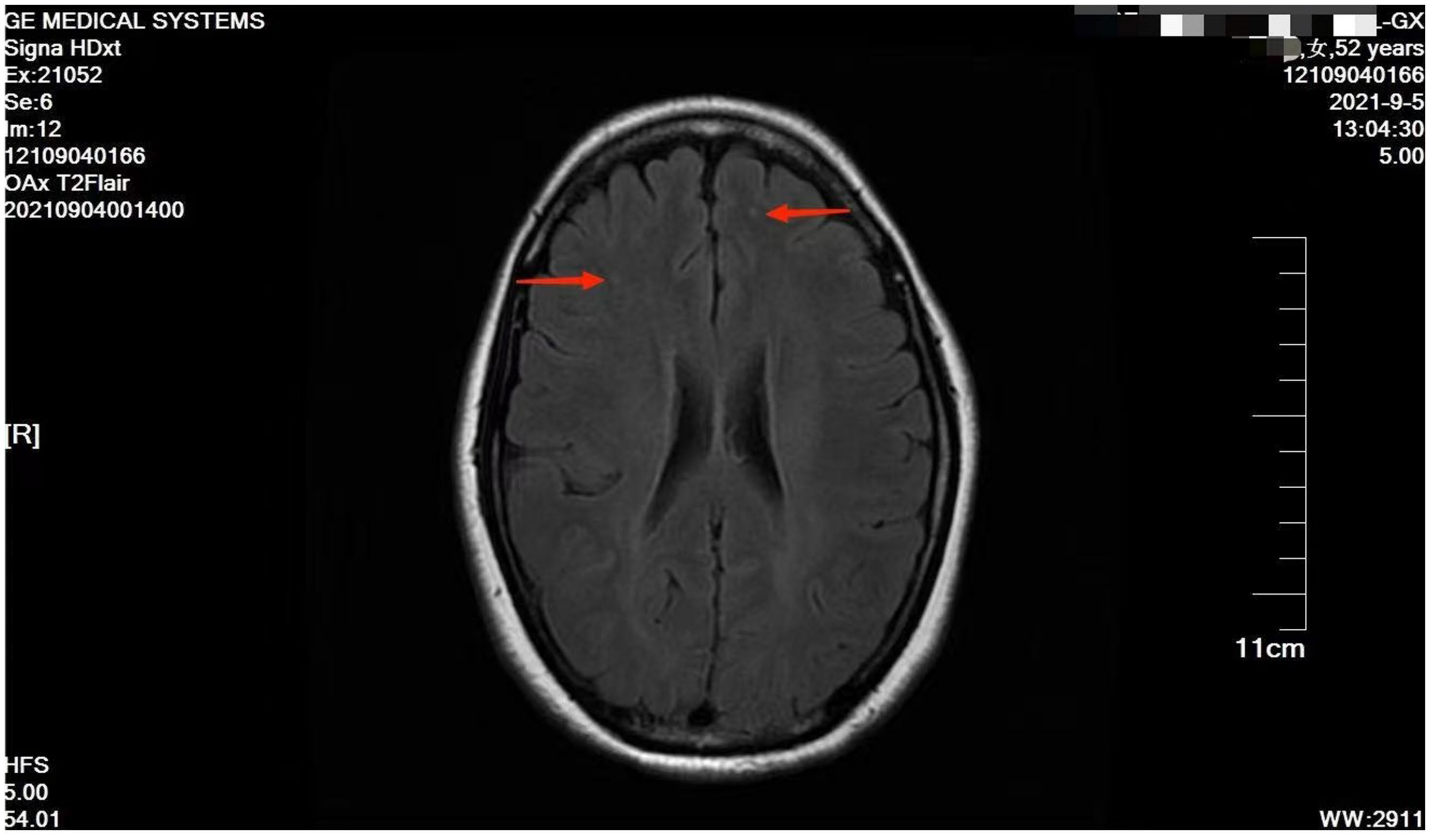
MRI T2 flare showed bilateral frontal lobe chronic small ischemic focus.
